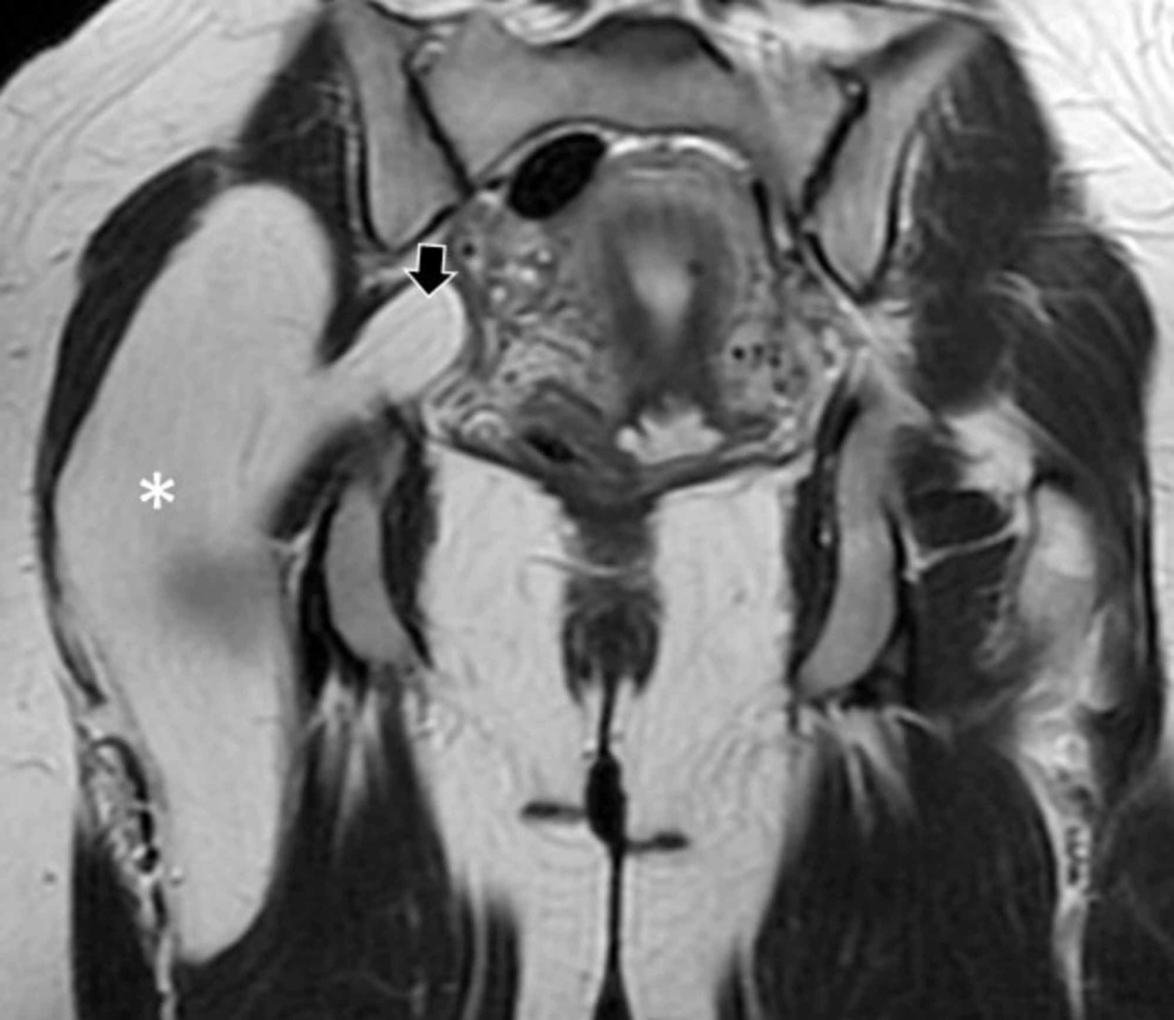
Preoperative magnetic resonance imaging (MRI) of the tumor herniating through the sciatic foramen Coronal T2W image showing an isointense to the fat tissue mass. Arrow indicates the pelvic and asterisk the gluteal portion of the mass.
